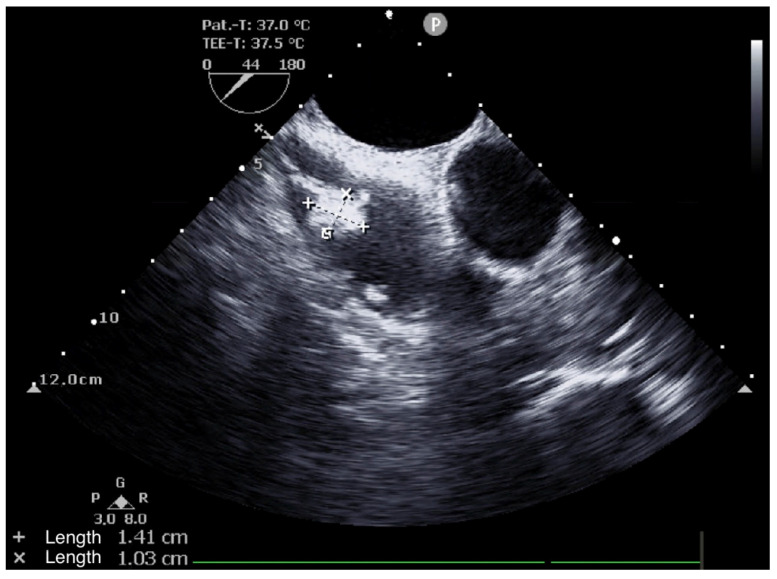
Pre-operative transesophageal echocardiogram (TEE), demonstrating the size of the right atrial (RA) mass.
